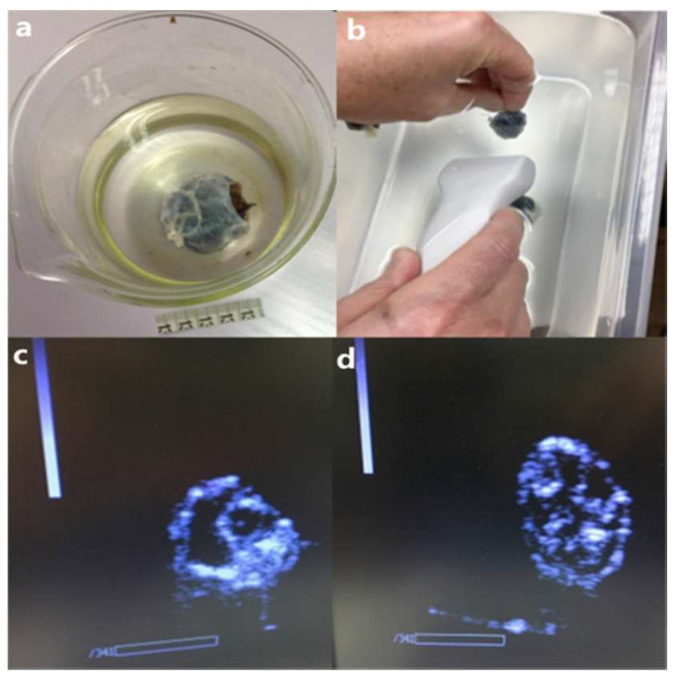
Ultrasonic examination of EndoXpand. (a) Intact black fungus covered by the outer membrane of casing. The corn silk ligature at one end had at this point degraded and broke. This released the black fungus pieces to the surrounding fluid. Since the individual pieces are of much smaller size, they will be able to pass the pylorus in humans without causing intestinal obstruction. (b) The testing environment for ultrasound experiments. (c) Ultrasound images of EndoXpand capsules with intact black fungus and granular black fungus (d).
